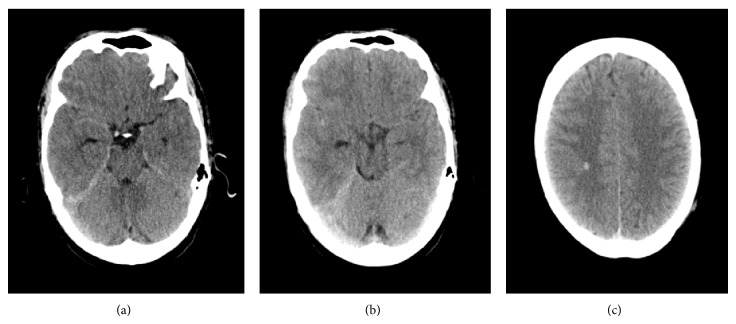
CT. ((a), (b)) Right frontoparietal subarachnoid hemorrhage. (c) Right parietal hematoma measuring 8 mm in diameter.
